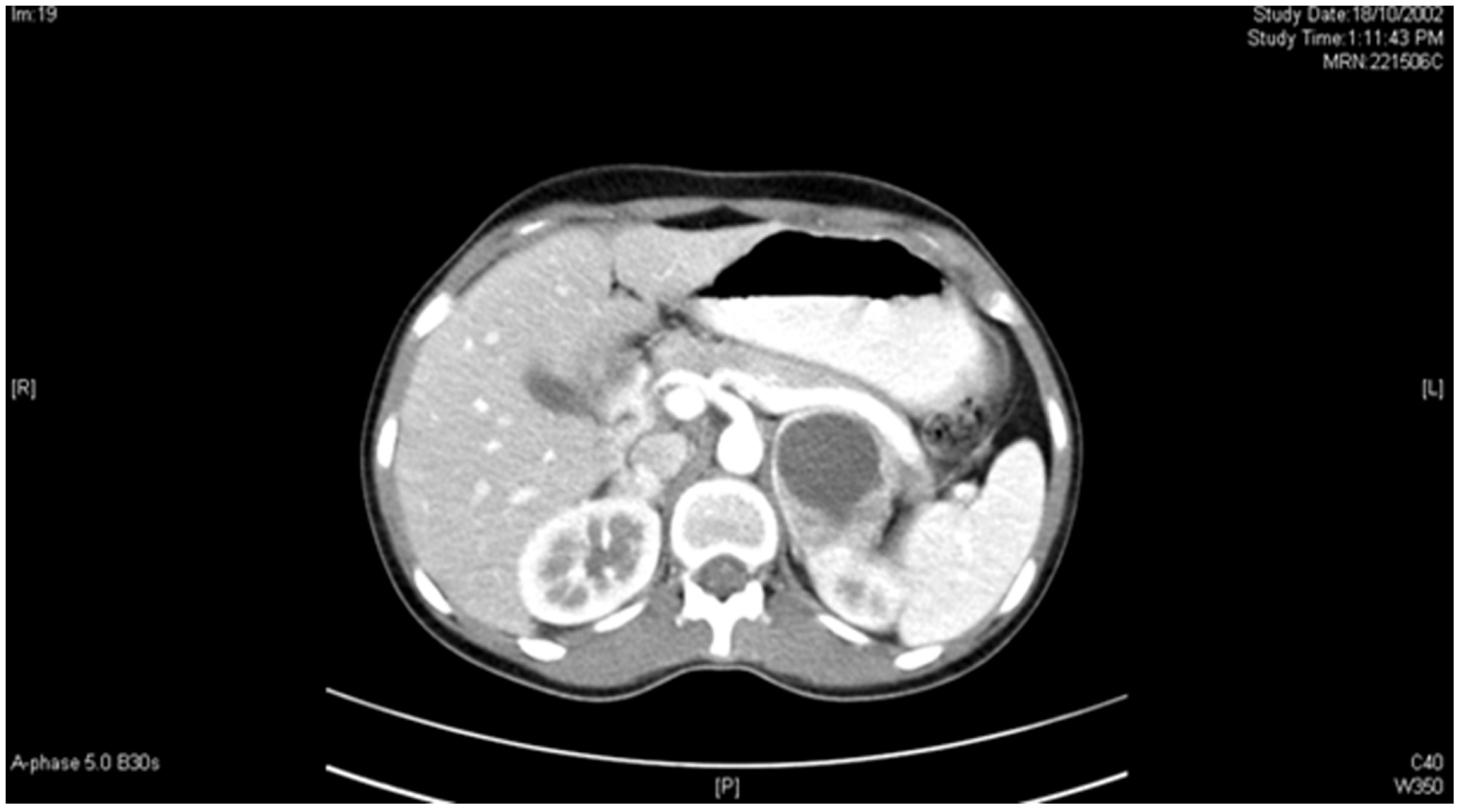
CT scan showing bilateral adrenal tumors.
